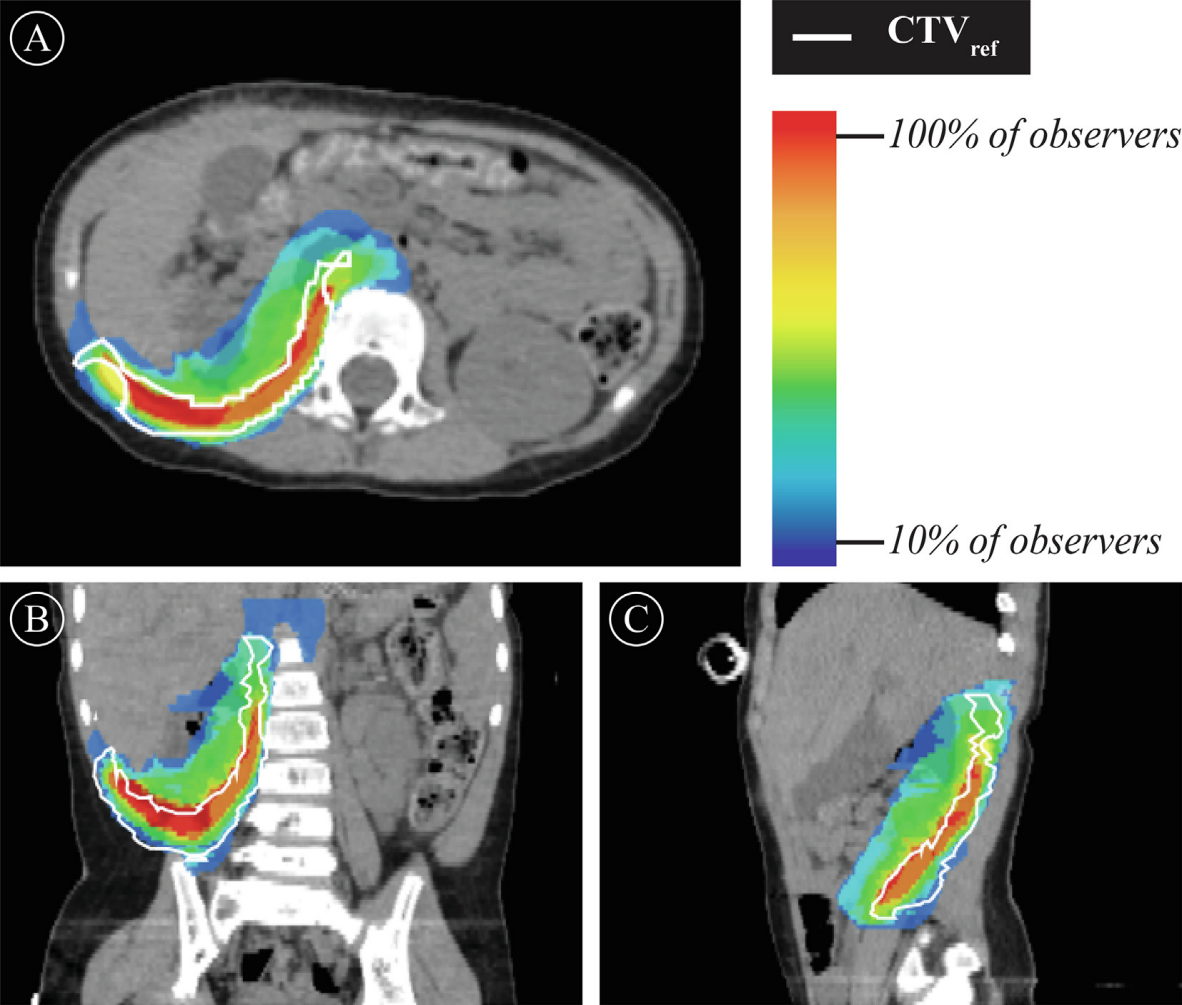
Count map of case 6 (female 2 years old, stage II WT-HR): the degree of agreement between the CTV_part_ (n = 9/10), alongside the CTV_ref_ (n = 1). For a total of 9/10 participants, overlap between the CTV_part_ for case 6 alongside the CTV_ref_ (*white*) is shown on the axial (3A), coronal (3B) and sagittal (3C) postoperative CT. Red and blue zones depict 100% and 10% agreement between participants only, respectively. *Abbreviations:* WT, Wilms’ tumor; HR, High-Risk; CTV, Clinical Target Volume. (For interpretation of the references to colour in this figure legend, the reader is referred to the web version of this article.)
